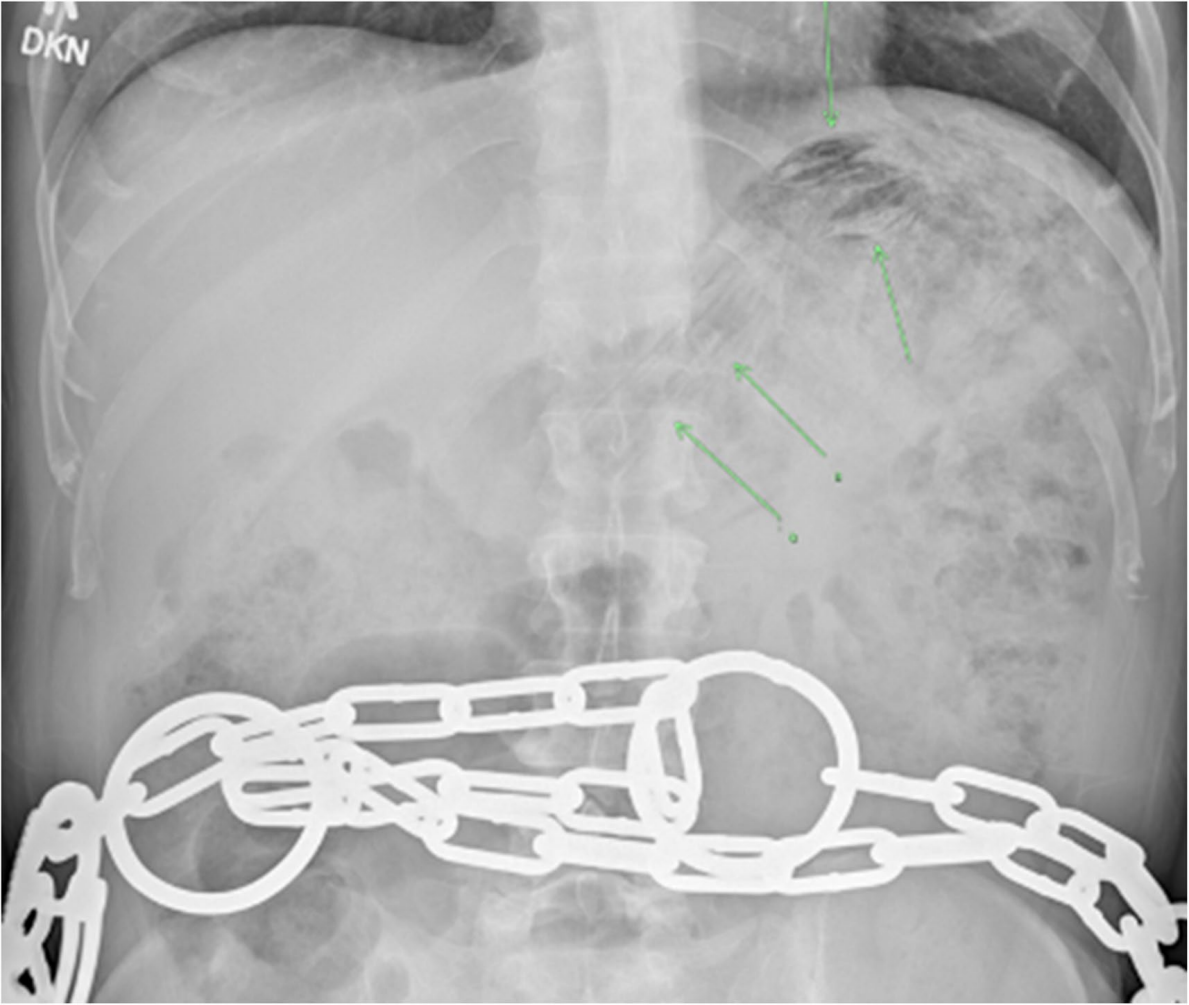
51-year-old male with past medical history of schizoaffective and schizotypal personality disorder and multiple prior foreign body ingestions. Abdominal radiograph shows multiple regular radiolucencies projecting over the gastric fundus and body in the left upper abdominal quadrant (green arrows), concerning for a radiolucent foreign body. This was initially missed due to the small difference in density between soft tissue and plastic bags. Upper GI endoscopy found multiple plastic bags, which were successfully removed
